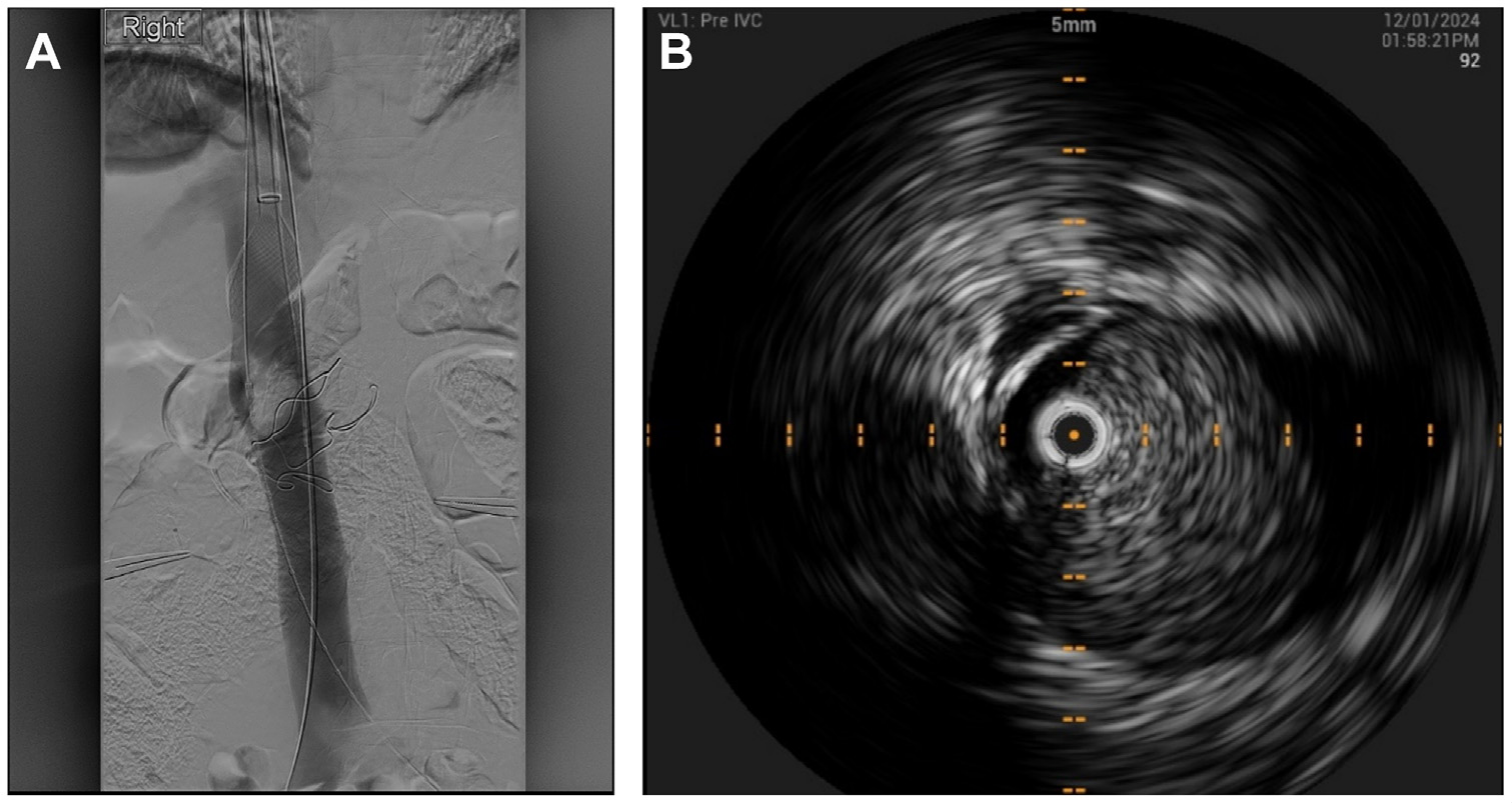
A, Digital substraction venography demonstrating a large immobile intraluminal mass in the pararenal inferior vena cava (*IVC*). There is no evidence of a distal or proximal filling defect, and it does not appear to invade the IVC wall. B, Intravenous ultrasound (*IVUS*) of the pararenal IVC demonstrating a markedly narrowed caval lumen with a large luminal mass causing near occlusion.
